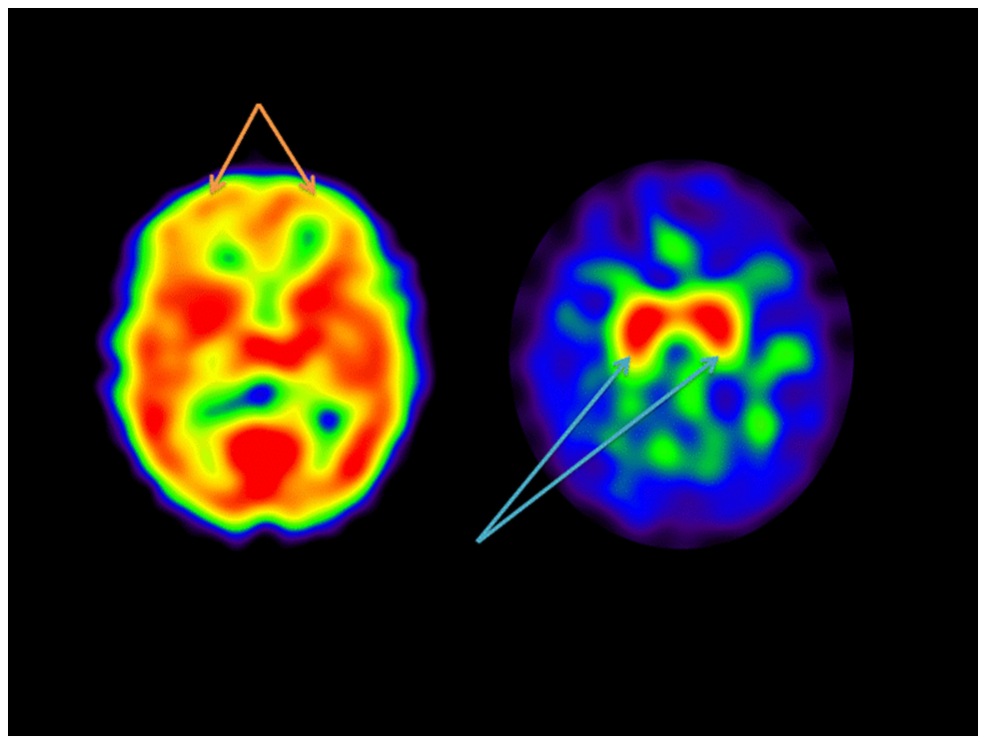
^99m^Tc Exametazime and ^123^I Ioflupane SPECT studies in a 61 year old male subject with multiple prior TBIs presenting with headache and memory loss. Note the markedly decreased CBF primarily in the frontal lobes bilaterally (yellow arrows). However, this same patient had robust DAT binding in the striatum (blue arrows) with no significant abnormalities.
